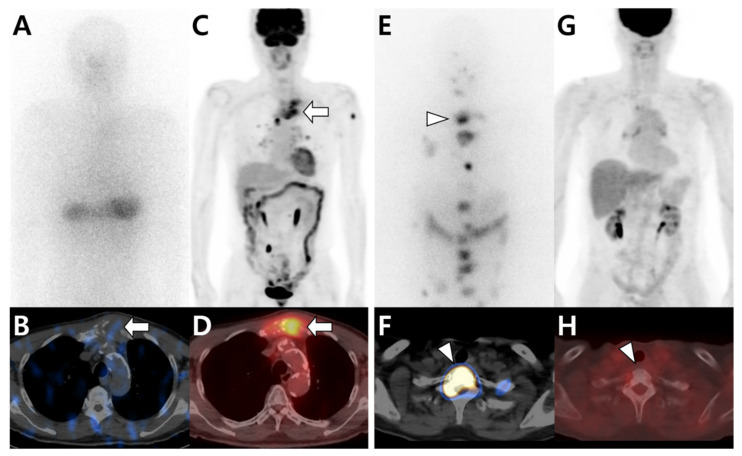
Different tracer avidities in bone metastases from papillary thyroid carcinoma (PTC) according to differentiation status. (A–D) A 79-year-old male patient with PTC. ^131^I whole-body scan (A) and single-photon emission computed tomography (SPECT)/computed tomography (CT) (B) show little iodine uptake in metastatic lesions located in the sternum (arrow). In contrast, ^18^F-fluorodeoxyglucose (^18^F-FDG) positron emission tomography (PET)/CT (C,D) shows intense FDG uptake in the sternum (arrow). (E–H) A 66-year-old female patient with PTC. ^131^I whole-body scan (E) and SPECT/CT (F) show multiple iodine uptake in metastatic bone lesions, including those in the T1 vertebra (arrowhead). However, ^18^F-FDG PET/CT (G,H) shows no significant uptake at the same site.
